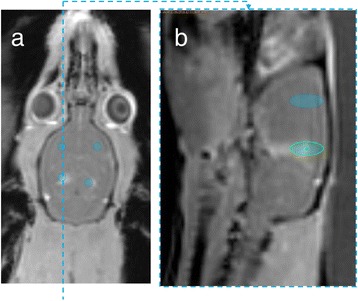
Axial (a) and sagittal (b) contrast-enhanced T1-weighted MRI scans indicating focal BBB disruption with intended targets overlaid in blue. From the top-left and clockwise in (a), the four targets had in situ pressures of 0.4, 0.5, 0.45, and 0.55 MPa
